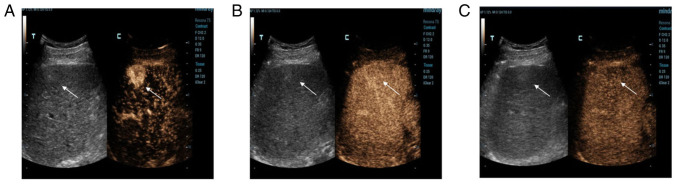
Contrast-enhanced ultrasound examination of liver segment 6 lesion. (A) The lesion (arrow) developed rapidly in the arterial phase. (B) The lesion (arrow) continued to develop in the portal vein stage. (C) In the delayed phase, the lesion (arrow) regressed slowly.
